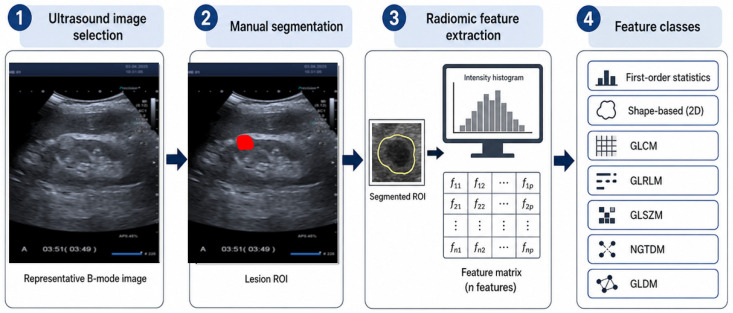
Radiomic workflow for B-mode ultrasound analysis of small hyperechoic renal masses. Representative B-mode images were selected, lesions were manually segmented to obtain a two-dimensional region of interest, and original radiomic features were extracted from the segmented lesion. Extracted feature classes included first-order statistics, two-dimensional shape-based features, gray-level co-occurrence matrix, gray-level run-length matrix, gray-level size-zone matrix, neighboring gray-tone difference matrix, and gray-level dependence matrix features.
